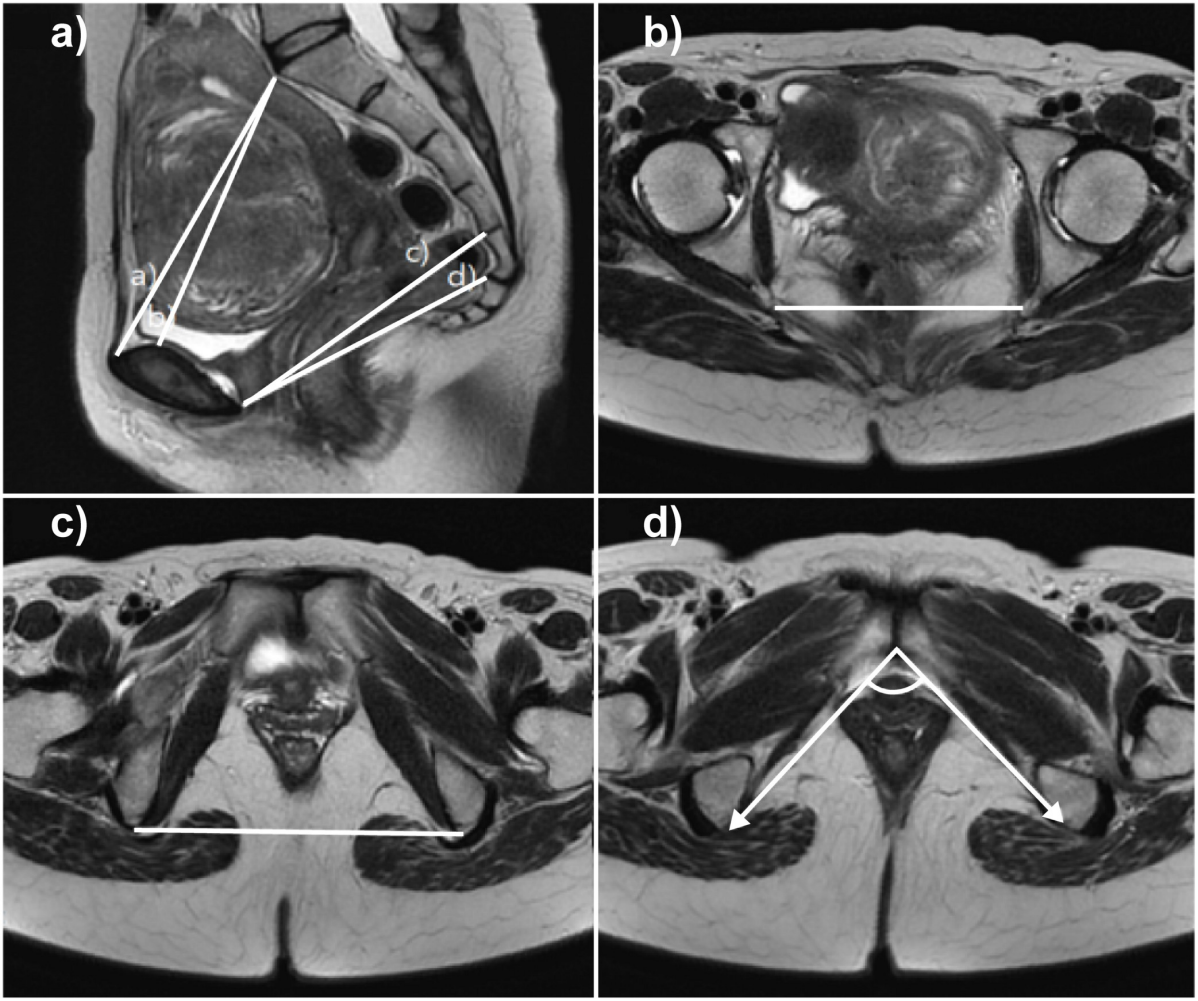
Pelvimetry parameters measured on magnetic resonance images of the female pelvis. (A) Sagittal view: (a) true conjugate (inlet-anteroposterior [AP] distance), (b) obstetric conjugate, (c) mid-AP distance, and (d) outlet-AP distance. (B) Interspinous distance in an axial plane at the level of the ischial spines. (C) Ischial tuberosity distance. (D) Pubic arch angle.
